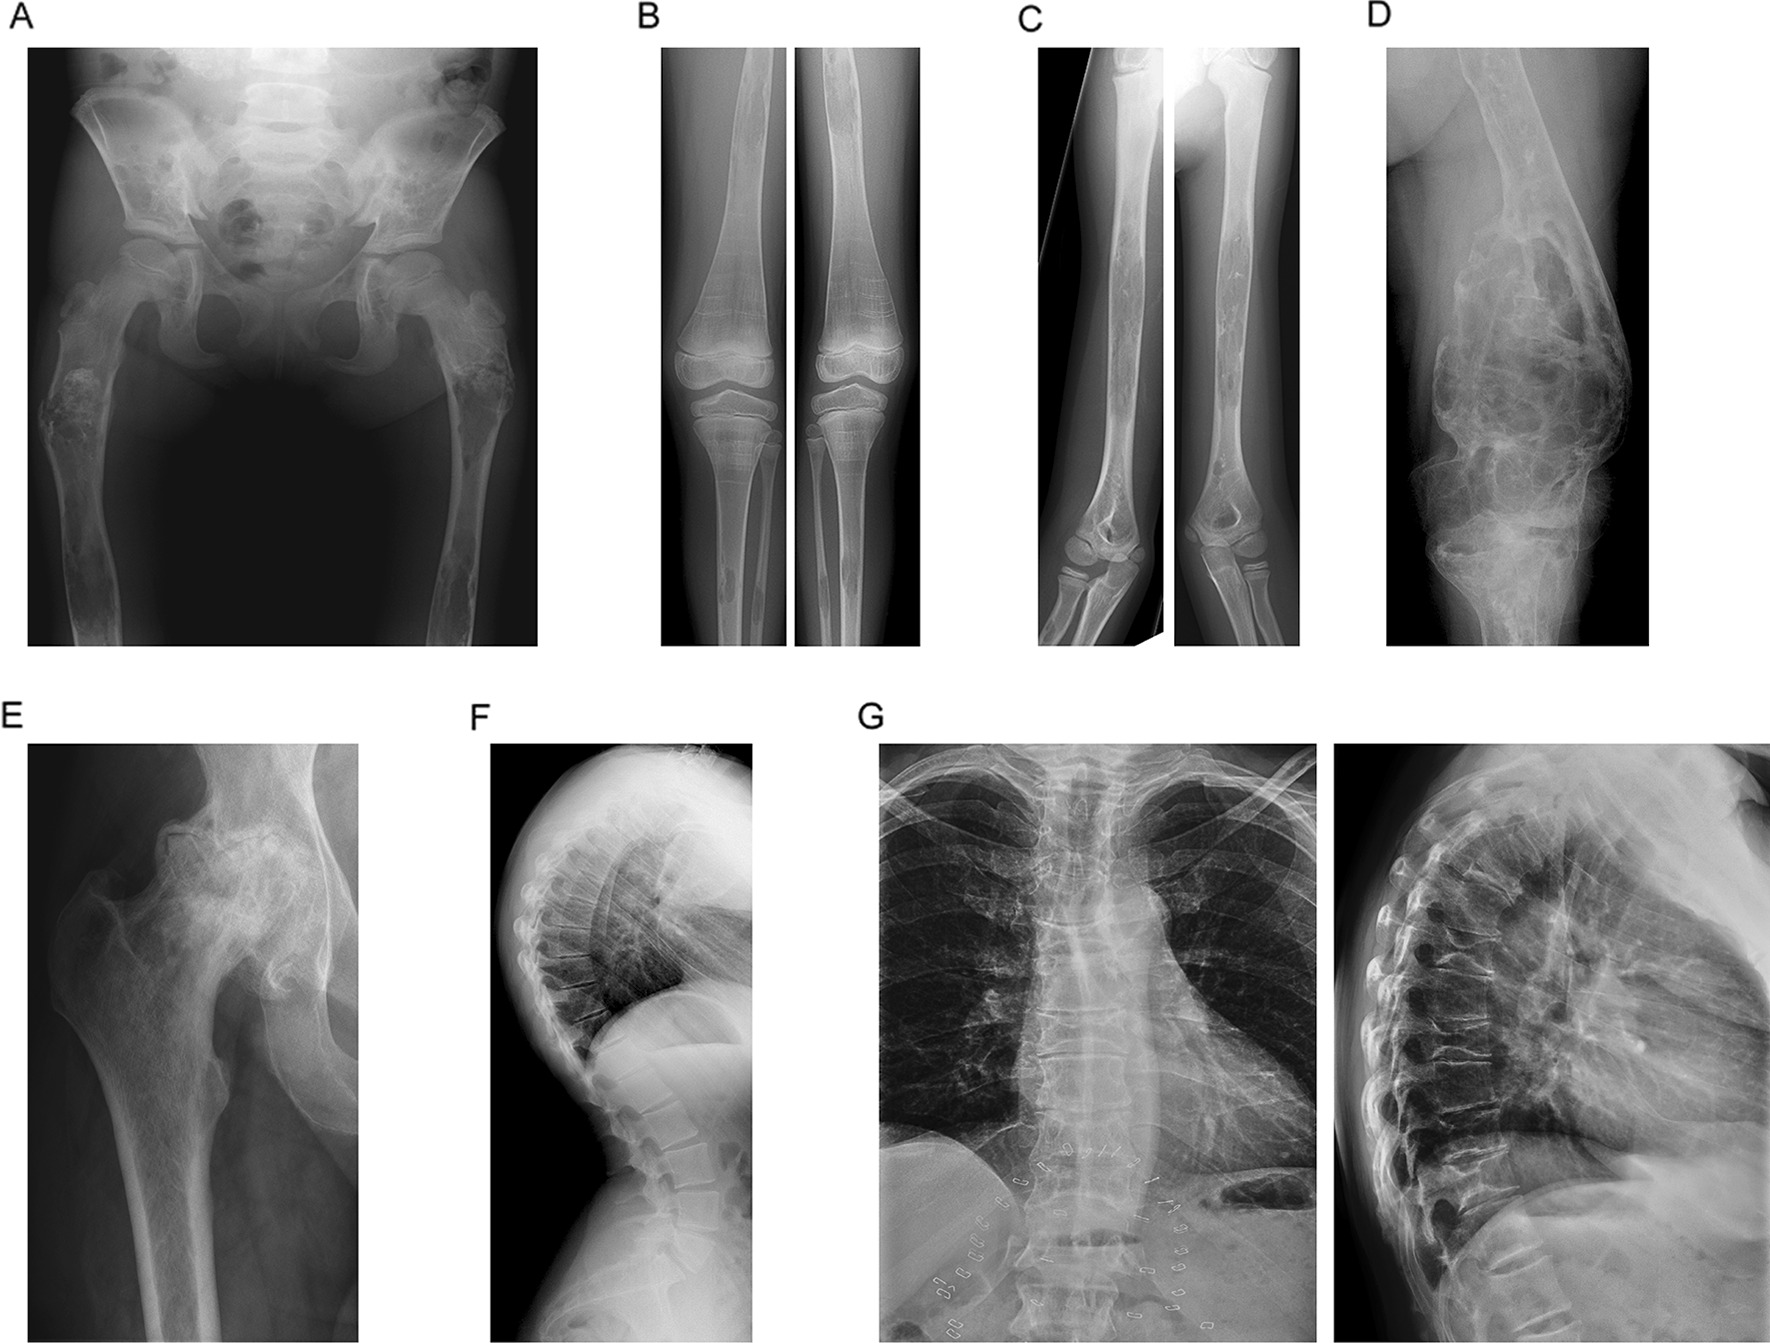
Radiographic imaging of skeletal manifestations in Gaucher disease. Generalized osteopaenia and lytic areas in iliac wings (A) and mid shafts of both femora (A, B), tibiae (B) and humeri (C). Pathological fractures both upper femora (A). Erlenmeyer flask deformity of both femurs, also known as metaphyseal flaring (B). Gross expansion and coarse trabeculation of the left femur (D). Extensive osteonecrosis of the right femoral head, which shows marked flattening with complete loss of joint space (E). Prominent thoracic kyphosis and exaggerated lumbar lordosis with minimal lumbar curve (F). H-shaped vertebrae of the thoracic spine consistent with osteonecrosis (G)
